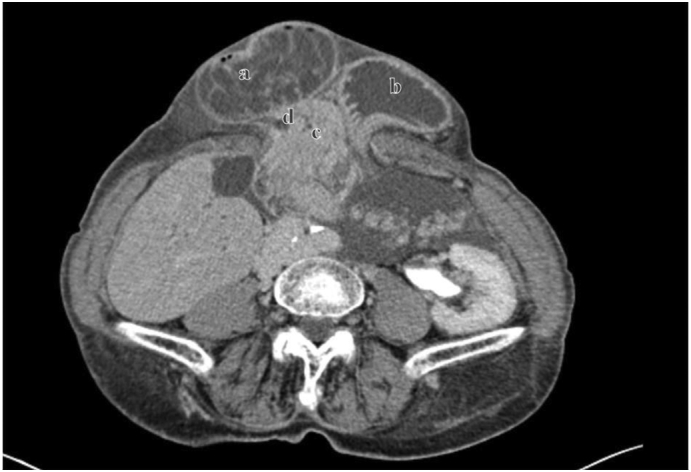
CT images. a: Duodenum; b: stomach; c: head of pancreas; d: choledochus.
